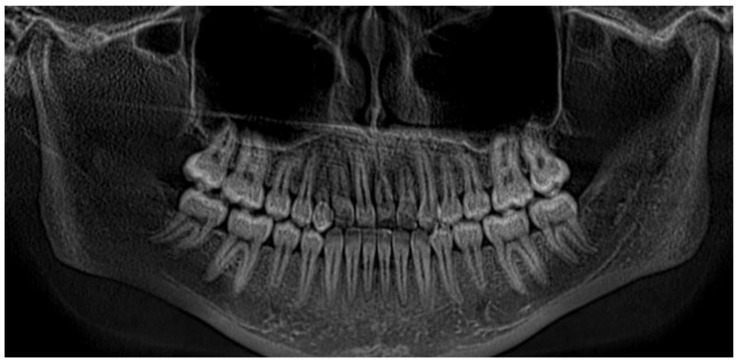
Final x-ray unveiling the position of tooth #13 in the site of tooth #11.
